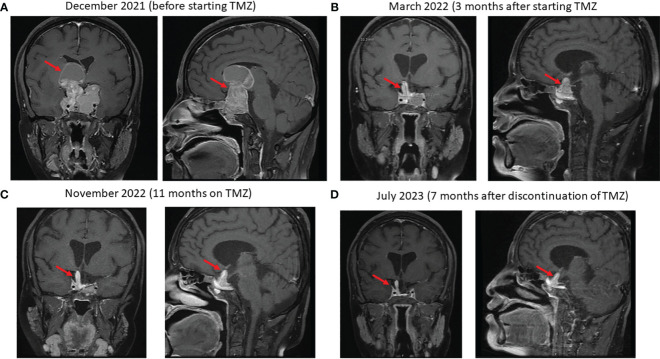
Sequential coronal and sagittal enhanced T1-weighted MR images showing the changes in the size of the macroprolactinoma (arrows) after starting temozolomide (TMZ): (A) at baseline just before starting TMZ, (B) 4 months later, (C) after 11 months on TMZ and (D) 7 months after discontinuation of TMZ.
